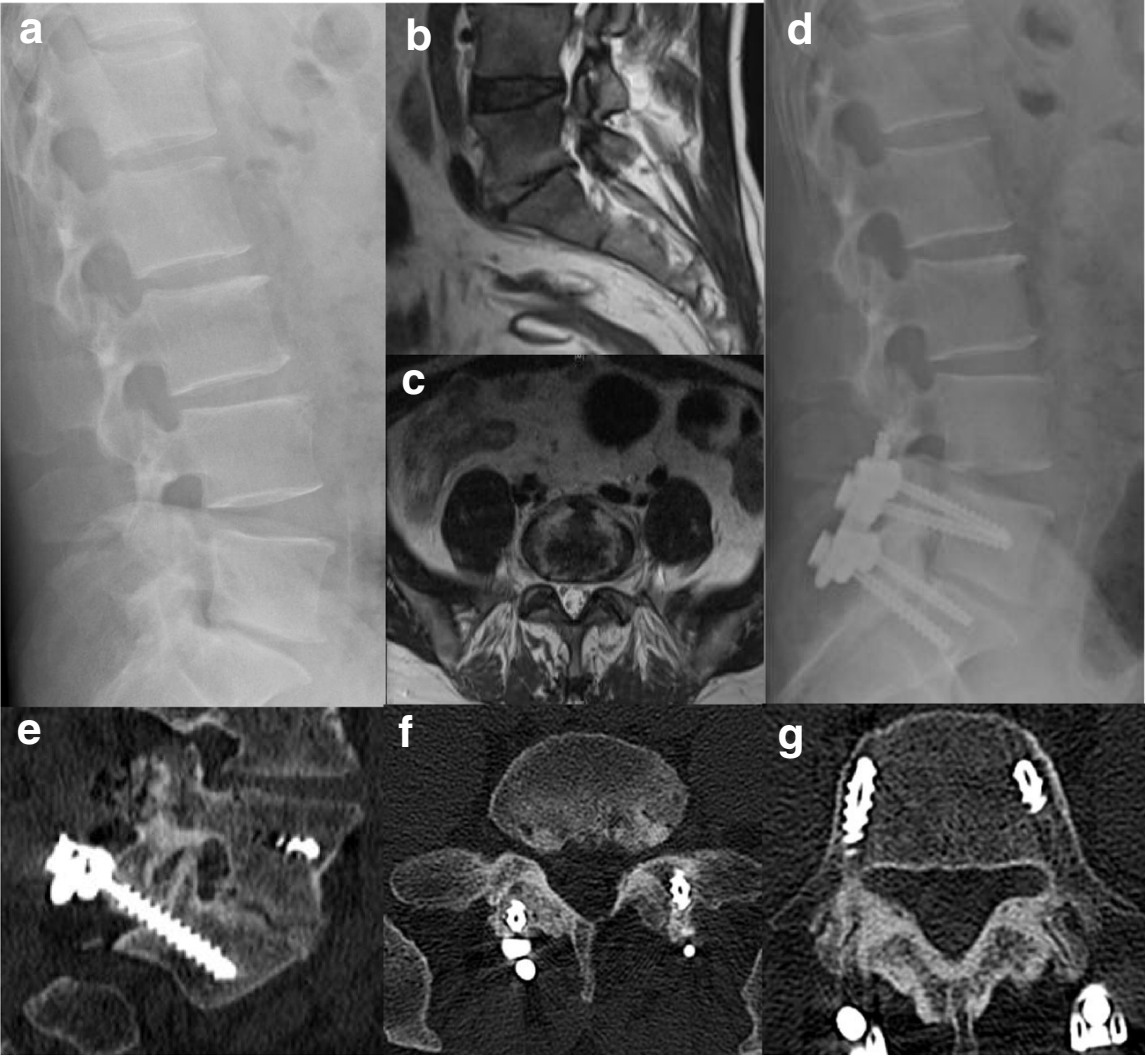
Exemplary case of a 47-year-old male presenting with immobilizing low back pain (VAS 5/10), fever and elevated procalcitonin, ESR, and CRP level. X-ray upon admission showed narrowing L5-S1 disc space with endplate destruction (a). Sagittal (b) and axial (c) T2-weighted MR images demonstrated bony endplate lesion and purulent mass at the L5/S1 level. Post-operative X-ray at 3 months (d) and spine computed tomography demonstrated bony fusion on the sagittal view at 33 months post-operatively (e). The canal was adequately debrided and decompressed on the axial view (f) with no screw loosening (g)
